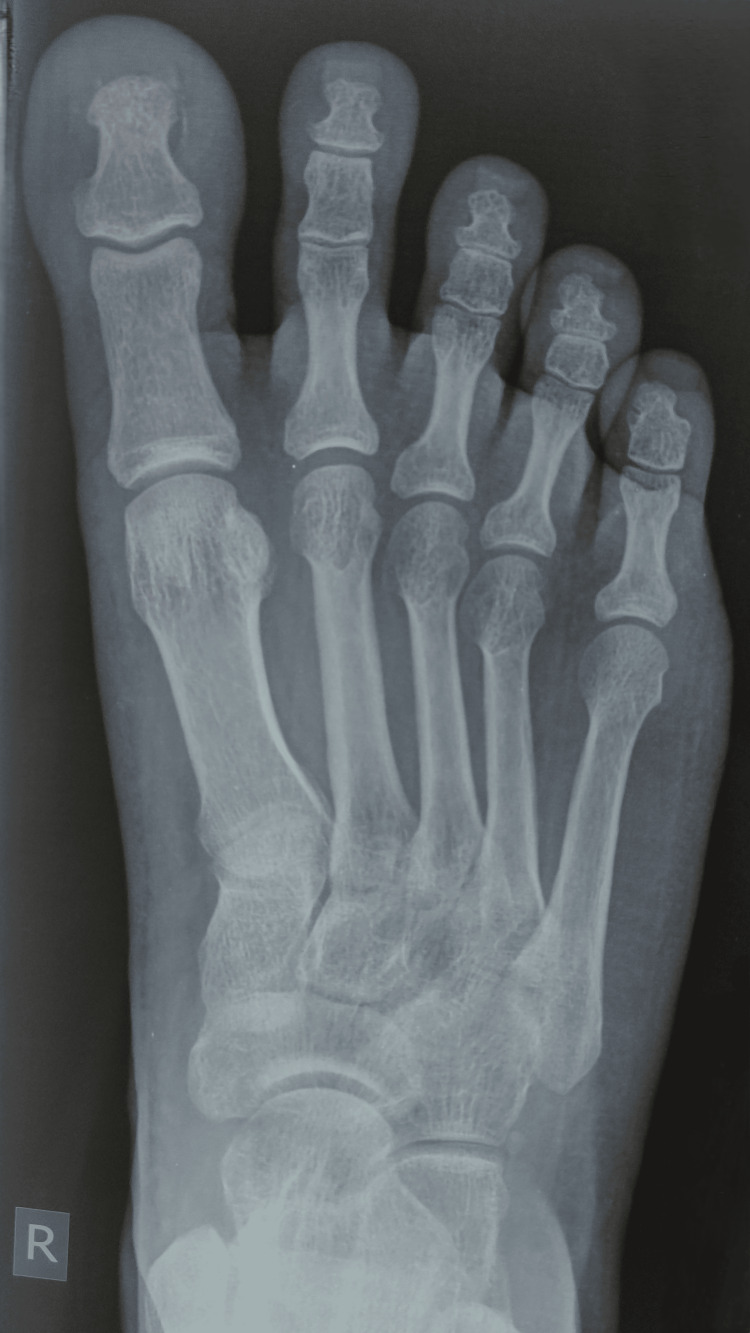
X-ray of the patient's right foot exhibiting no bony abnormalities
